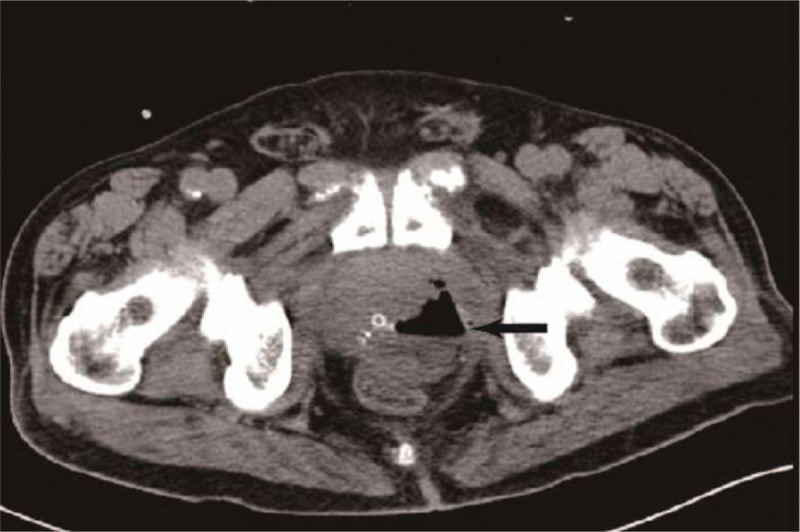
Pelvic CT revealed a collection of gas and purulent exudates in the prostate gland (arrow) on day 8 of admission (before aspiration).
